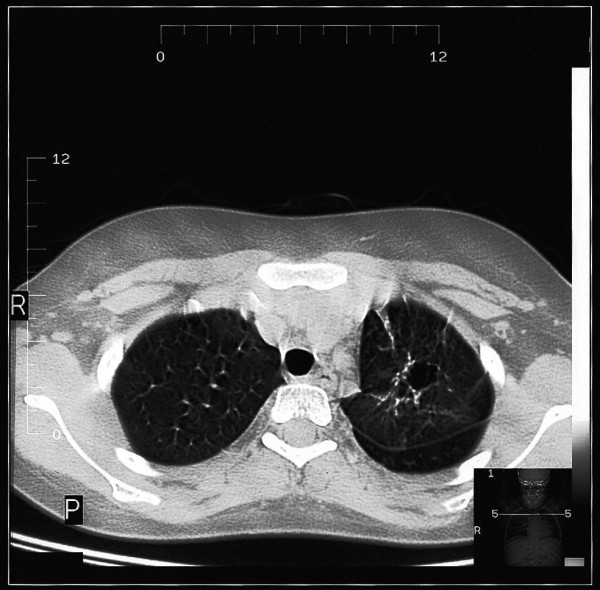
Fine nodules, interstitial infiltrations and a cystic lesion on chest high-resolution computed tomography (HRCT) in a child with HIES.
